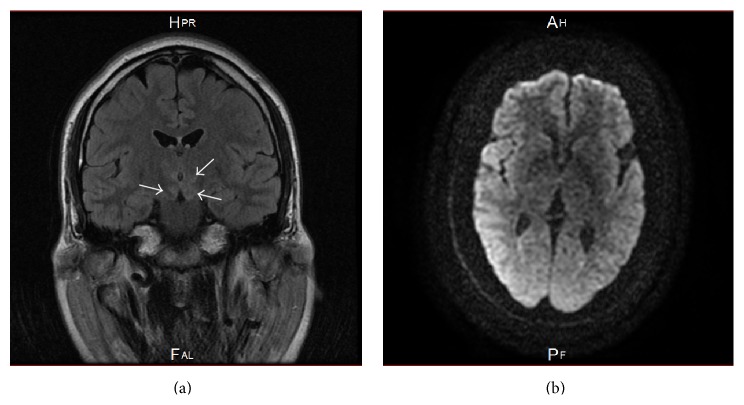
Brain MRI scans ten days after initiating the antibiotic therapy showing residual hyperintense signal alternations in the hypothalamus, in the left crura cerebri, and in the left thalamus (arrows) on the coronal FLAIR-T2 in (a) and complete regression of the signal abnormality on the axial diffusion weighted imaging DWI in (b).
